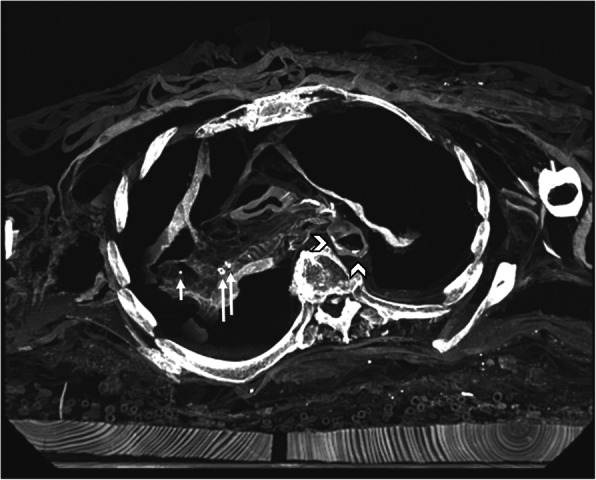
CT image of Ranke complex. CT image of Peder Winstrup’s chest in a slightly angled axial plane with the short arrow showing a small calcified granuloma in the probable upper lobe of the collapsed right lung, and two approximately 5 mm calcifications in the right hilum together suggesting a Ranke complex and previous primary tuberculosis. The more lateral of the two hilar calcifications was extracted for further analysis. In addition, there are calcifications in the descending aorta proposing atherosclerosis (arrowhead)
